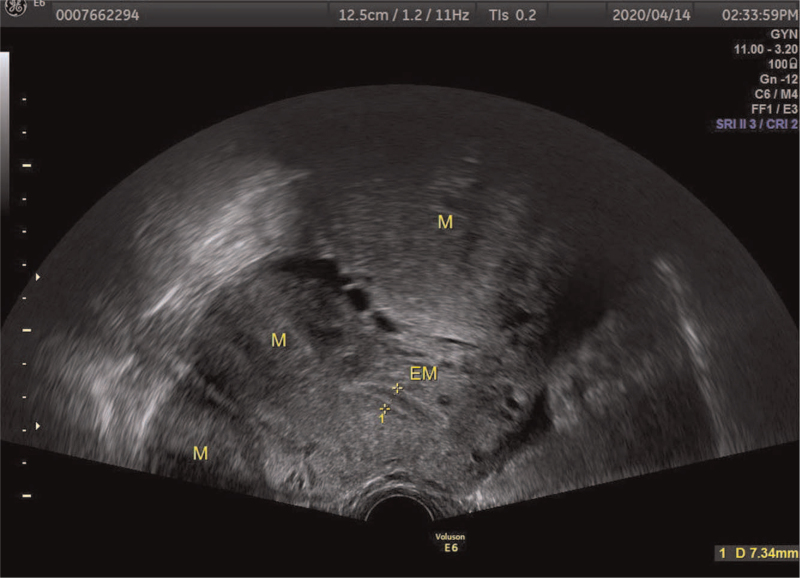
Multiple uterine leiomyomas by pelvic ultrasound. The size of the largest one was approximately 98 × 85 × 113 mm.
